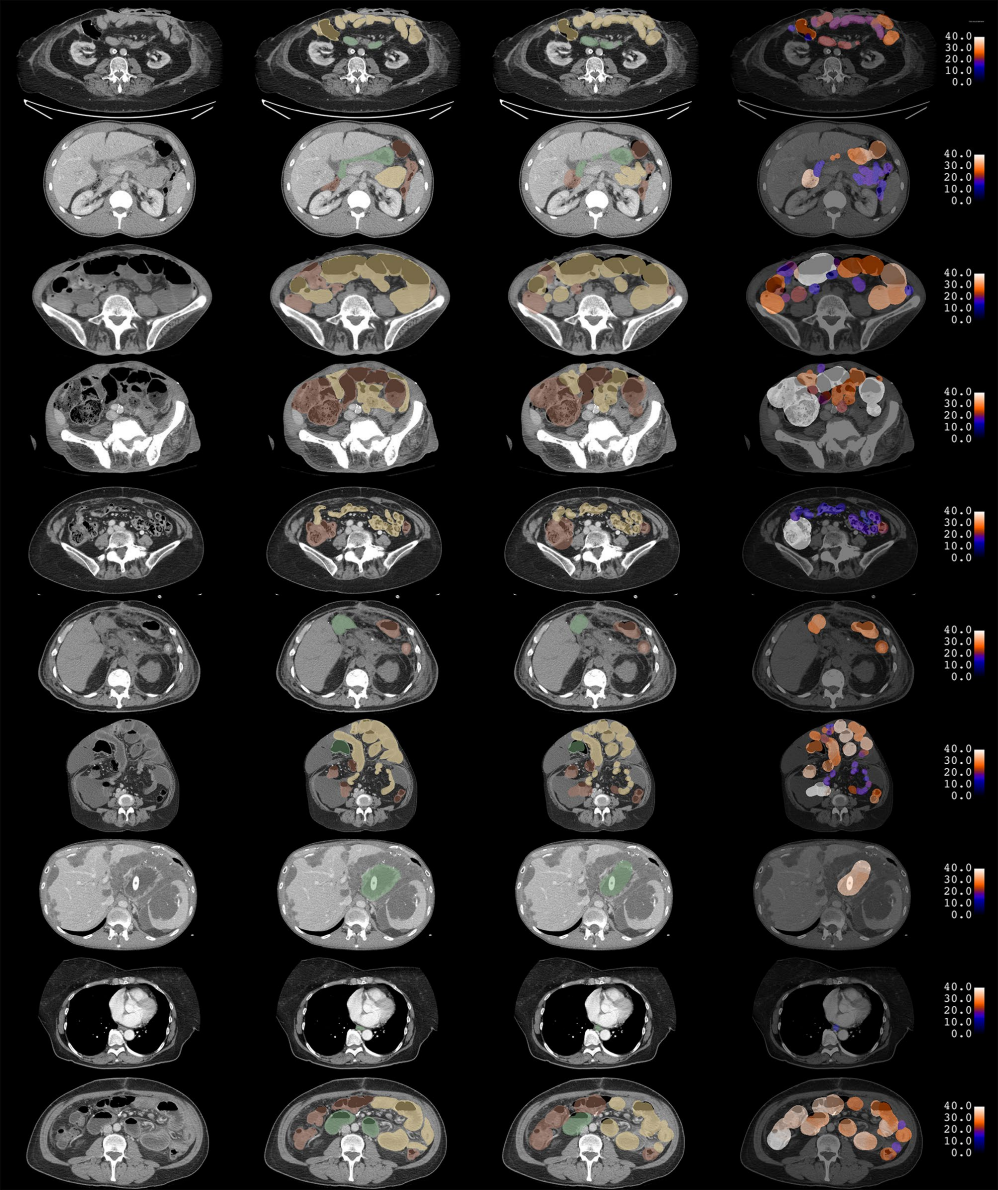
Examples of manual and visual annotation. Each row corresponds to a single slice of a CT scan for 10 different subjects. The CT scan is shown in the first column. Manual segmentations are shown in the second column. Segmentations from visual annotation are shown in the third column. In both segmentations, red, yellow, and green correspond to foregut, midgut, and hindgut, respectively. Diameter maps from visual annotation are shown in the fourth column. Diameters are expressed in units of millimeters. Manual diameter measurements are not shown
